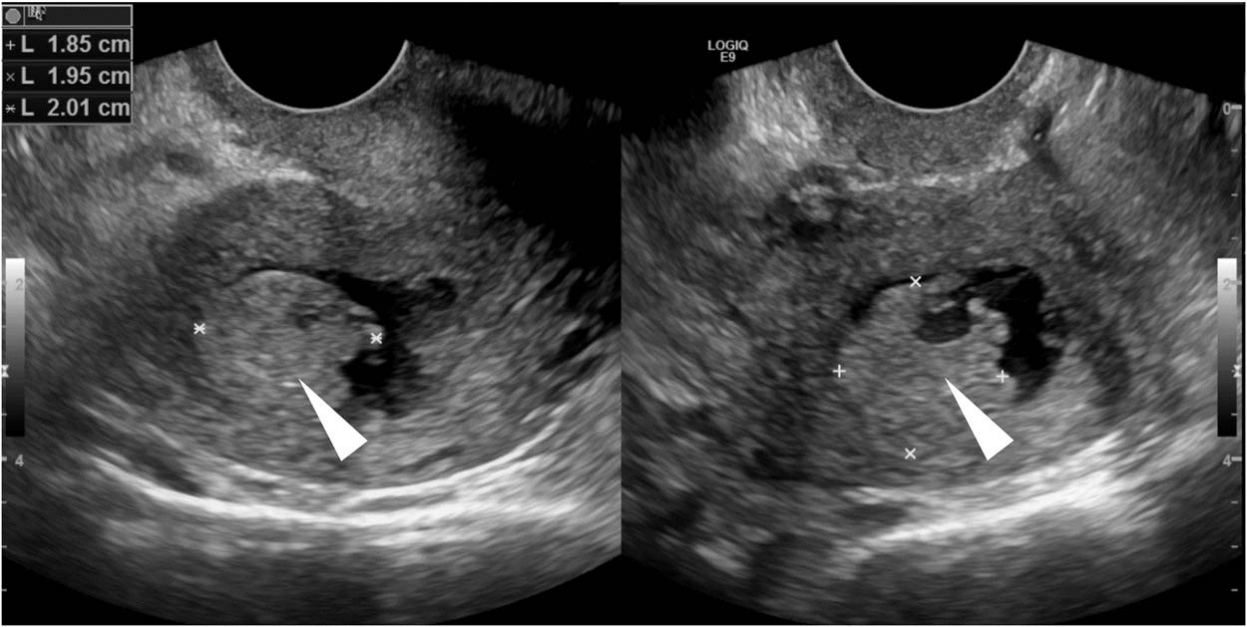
Transvaginal pelvic ultrasound demonstrates an approximately 20 mm echogenic endometrial mass (arrowhead) with irregular margins. The mass appears to invade the myometrium.
